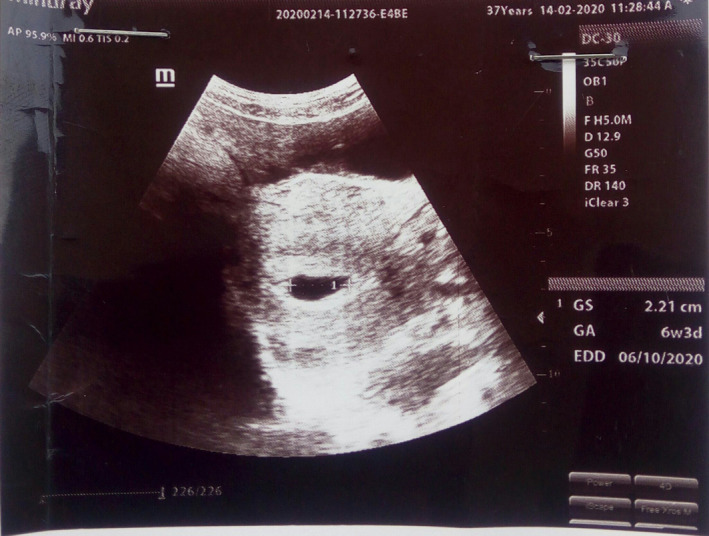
Obstetric ultrasound scan taken on 14th February 2020. Gestational sac (GS) was 2.12 cm, and gestational age (GA) was 6 weeks and 3 days according to the scan. Expected date of delivery was 6th October 2020
